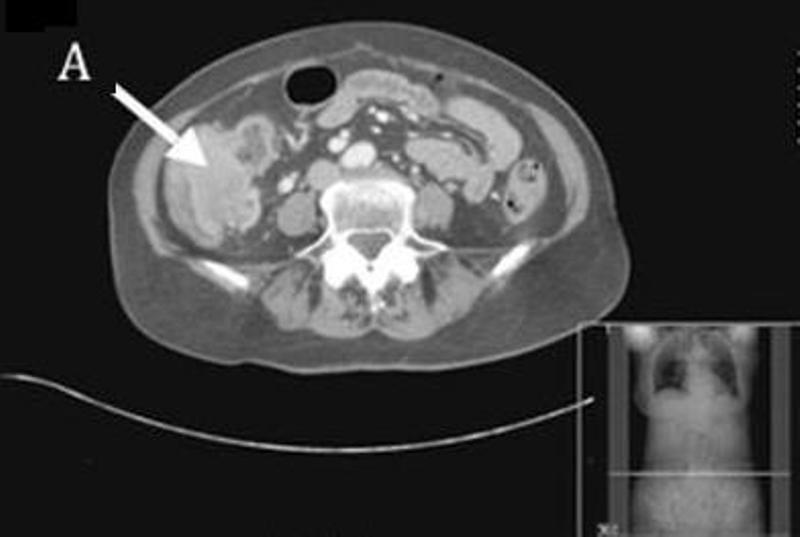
Computed tomography showing a recurrence of Merkel cell carcinoma in the caecum at 18 months after the initial diagnosis. There is a 6 × 4.3 cm soft-tissue mass seen in the region of the ileocecal valve (A) which extends into the lumen of the caecum. There are multiple abnormal lymph nodes measuring up to 2 cm within the ileocolic mesentery. There is nodularity and irregularity seen around the tumour extending into the pericolic fat suggestive of a local tumour infiltration.
